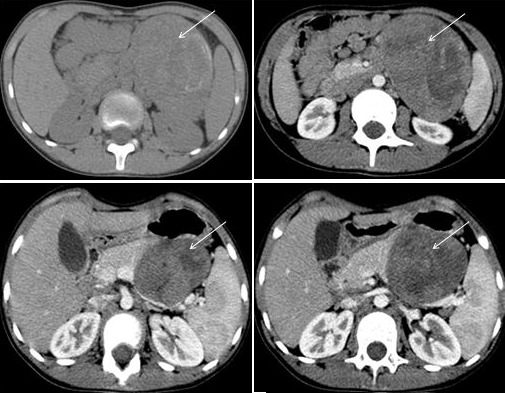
Computed tomography (CT) imaging identified a well-circumscribed mass located in the body and the tail of the pancreas (arrow). It was heterogeneous, containing cystic and solid portions, with peripheral calcifications. The solid portion had heterogenous enhancement after intravenous contrast administration, demonstrating some necrotic areas
